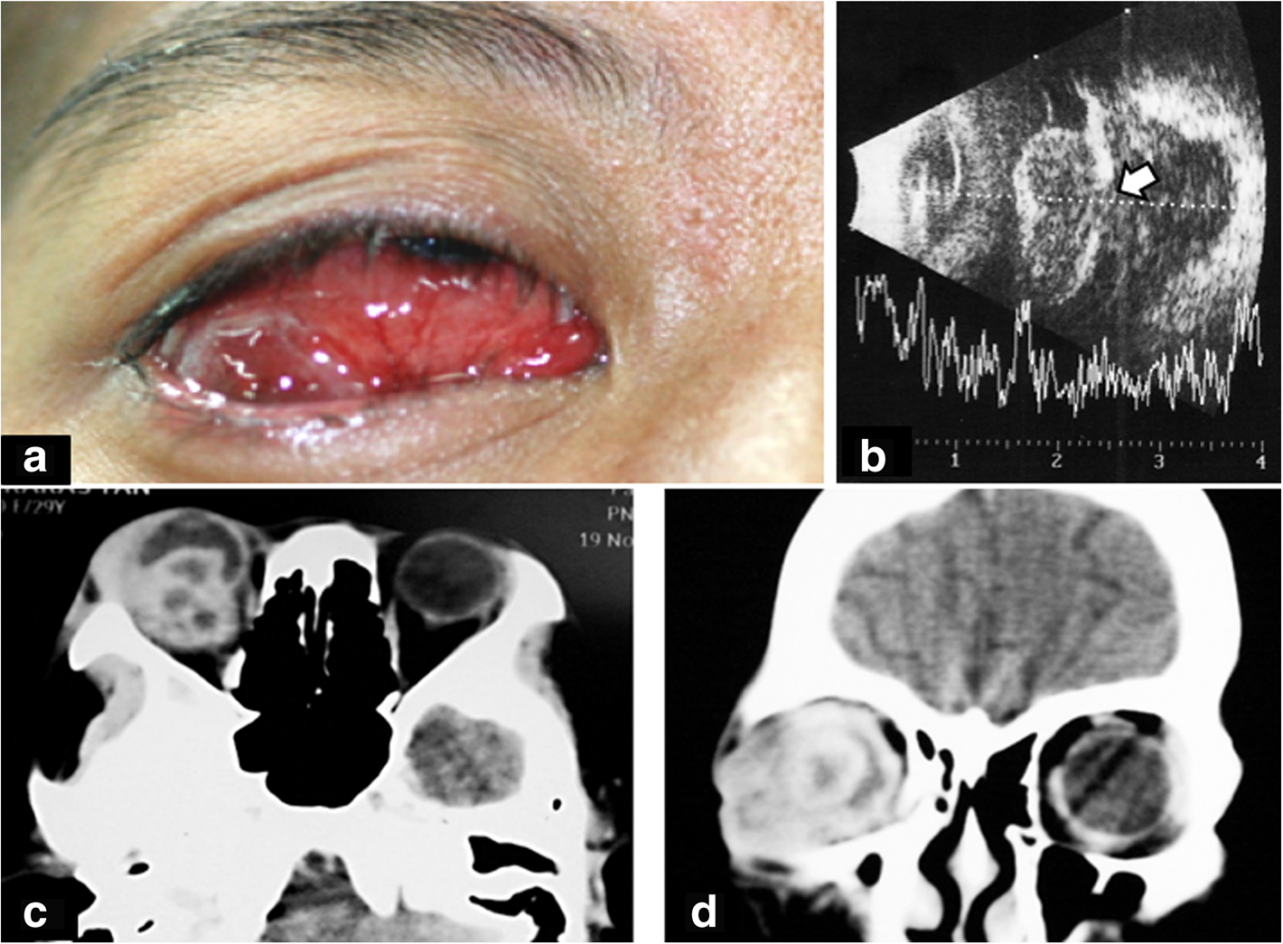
a A large subconjunctival abscess is shown in the right eye inferotemporally with purulent discharge and chemosis. b B-scan ultrasonogram of the right eye shows a large intraocular mass extending beyond the globe through a scleral defect (*arrow*) into the orbital cavity. c and d Orbital computed tomographic scan shows in more detail the conjoint intraocular and intraorbital multiloculated, multiple-ring, contrast-enhanced mass
